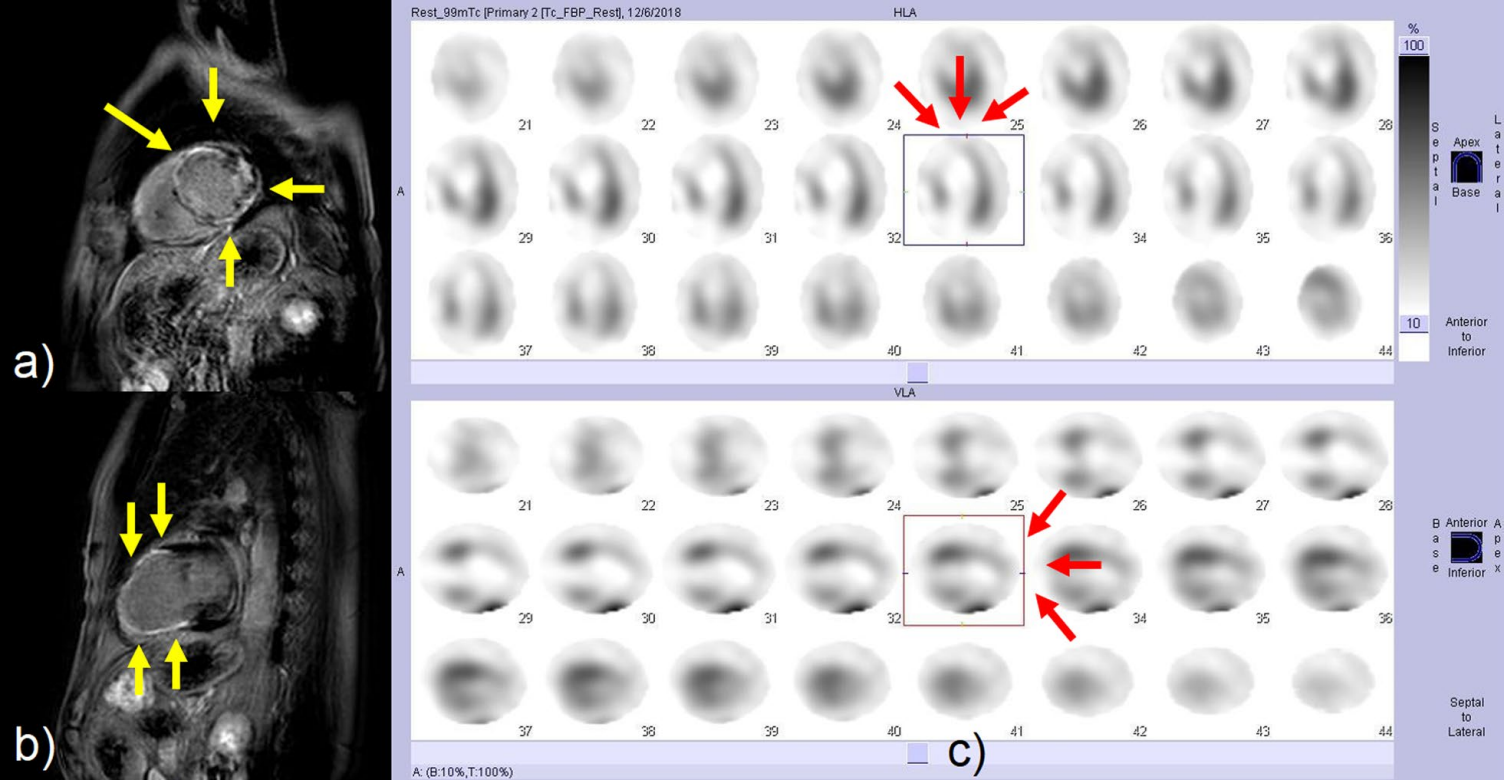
Cardiac magnetic resonance imaging (MRI) and myocardial scintigraphy in the chronic phase. Late gadolinium enhancement in the short axis (a) and two-chamber (b) views show diffuse fibrosis of the left ventricular wall especially in the inferior and anterior wall (yellow arrows). Decreased viability may be commonly observed on the anterior wall and septum of the left ventricular myocardium from the middle to the apex (red arrows) (c)
